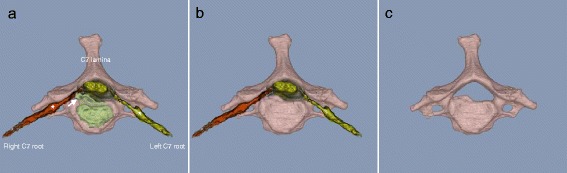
3D MR/CT fusion images showing compression of the right C7 nerve root by an extruded herniated disc at the pre-foraminal site at C6/7. Images show the C7 vertebra, C7 root and spinal root ganglion with (Panel a) and without (Panel b) the intervertebral disc and C7 vertebra alone (Panel c). The affected side is shown in red. The disc is shown in green. Asterisks indicate the ganglion and the arrow indicates the herniated disc.
